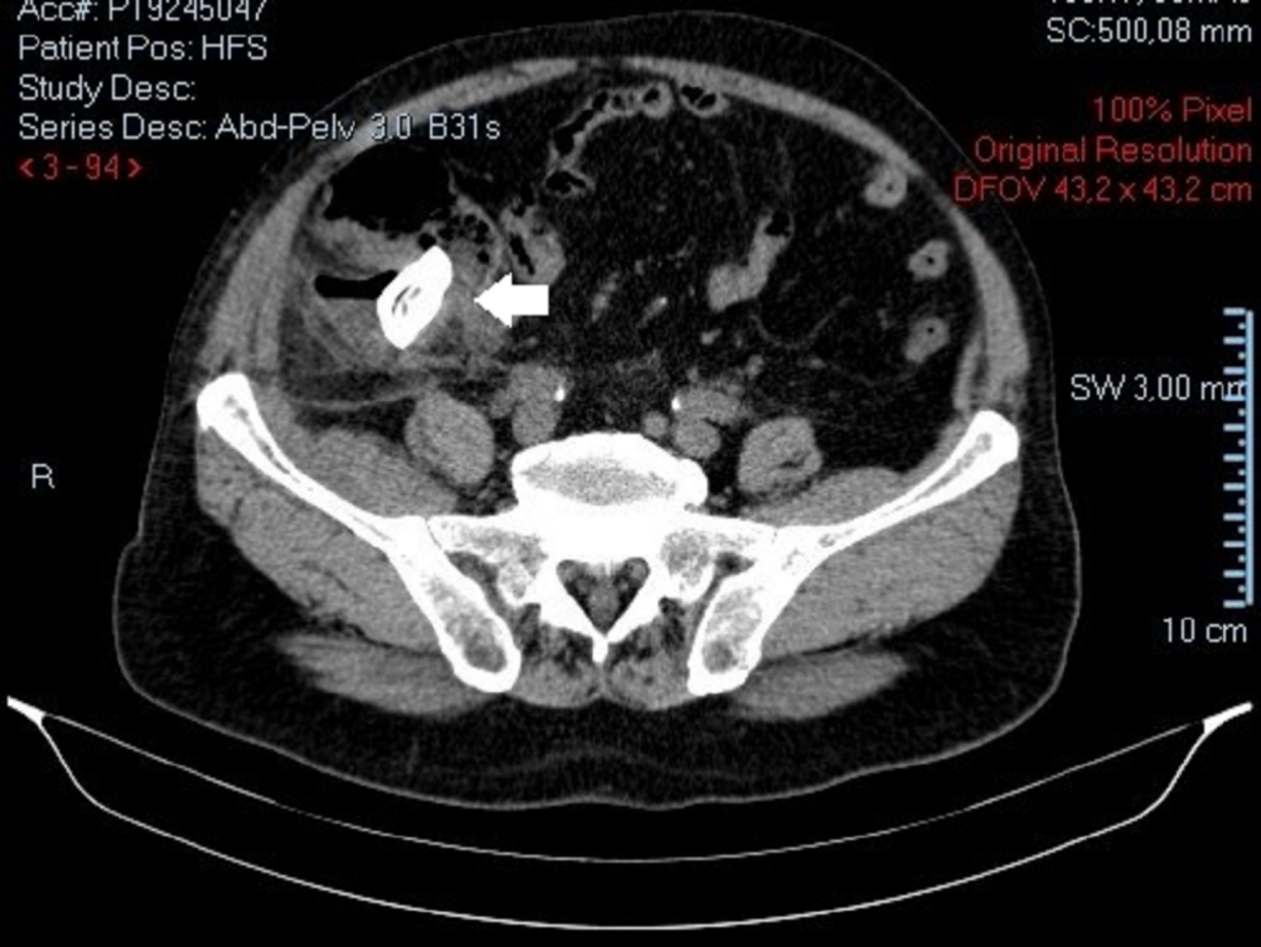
Abdominopelvic CT scan. Presence of a presumed "appendicolith" of 35 mm at the cecum near the base of the ileocecal appendix.
